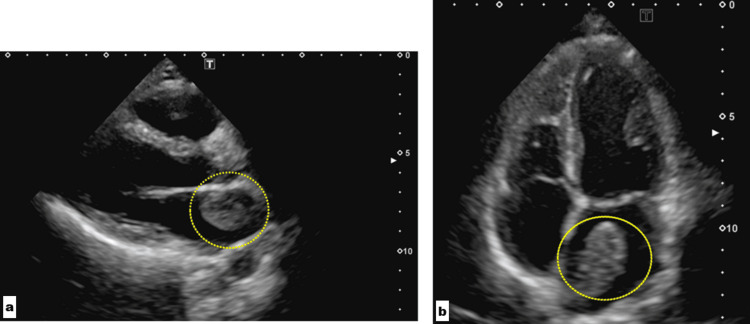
Echocardiographic image of the left atrium nodule (a, b) The mobile mass with heterogeneous internal echoes measuring 43 × 25 mm in the left atrium (inside circles)
